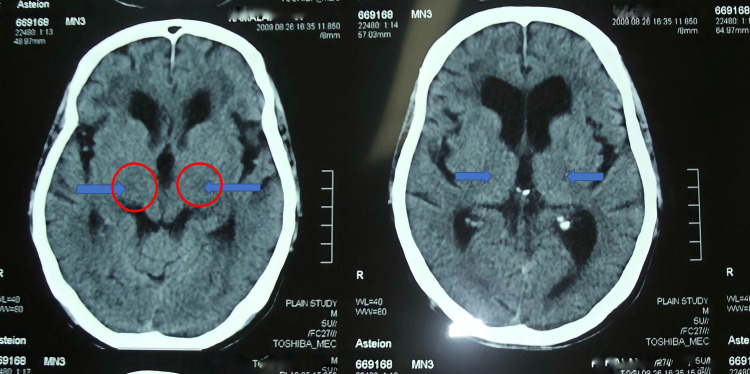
CT brain delineated an acute left peri-sylvian infarct and a chronic right internal capsular anterior limb infarct (shown in blue arrow and red circles).
